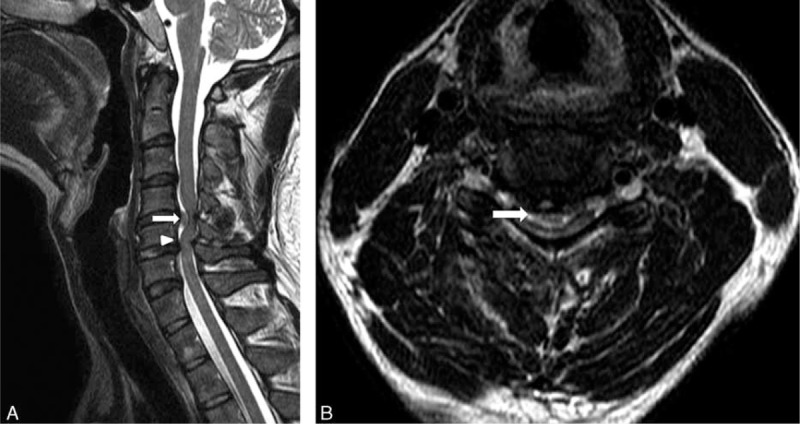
Preoperative MRI. (A) Sagittal T2-weighted image of the cervical spine revealed large disc herniation at C4–C5 (arrow) and C5–C6 with severe spinal cord compression. The “Y-sign” (arrow head) was found. (B) Axial T2-weighted image of the cervical spine revealed large, central disc herniation at C4–C5 with severe spinal cord compression and surrounding edema. A “halo” of CSF isointensity surrounding the herniated disc was also demonstrated (arrow). CSF = cerebrospinal fluid, MRI = magnetic resonance imaging.
